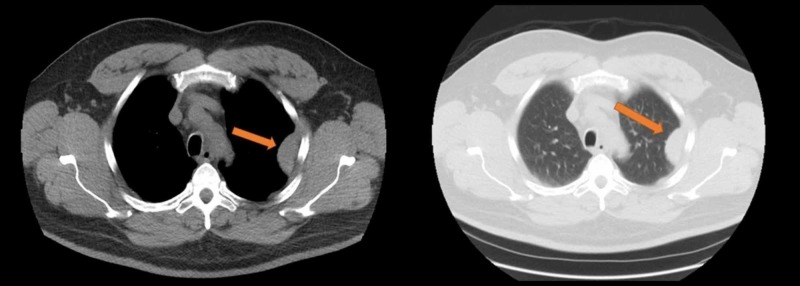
CT scan chest Computerized tomography (CT) scan of the chest showing a pleural-based left upper lobe mass (orange arrows).
